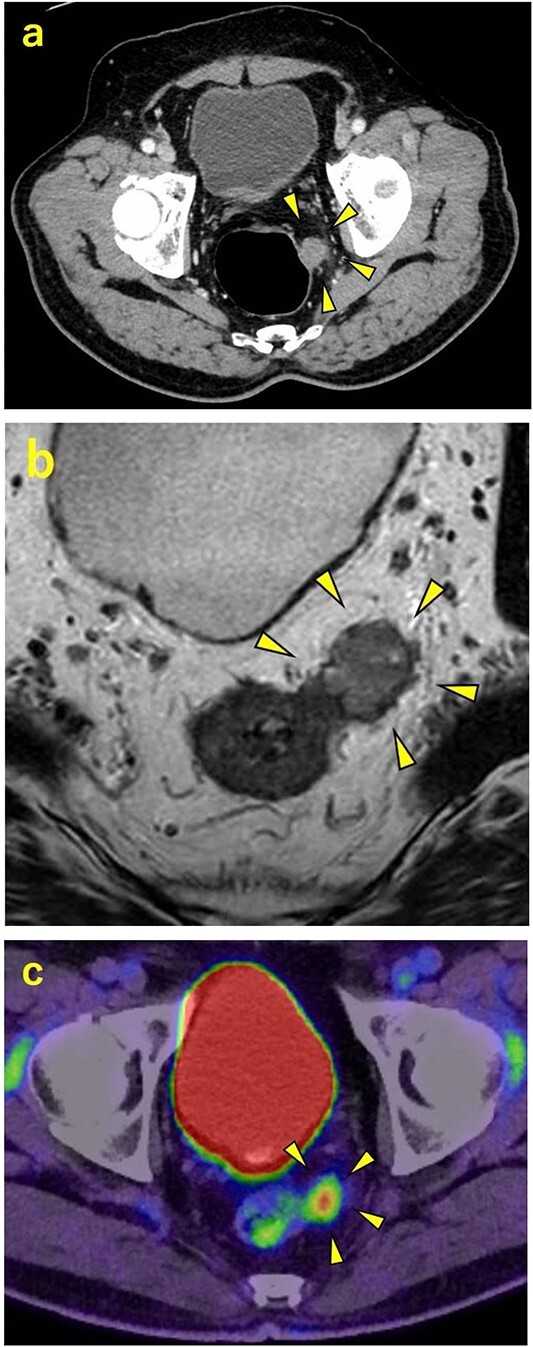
Contrast-enhanced CT, contrast-enhanced MRI, and PET. (a) CT showing a 20-mm large extramural growth-type mass on the left wall of the rectum, 9 cm from the anal verge. (b) MRI showing that the mass may have originated from the muscularis propria of the rectum. (c) PET showing an accumulation in the mass with a maximum standardized uptake value of 5.96.
